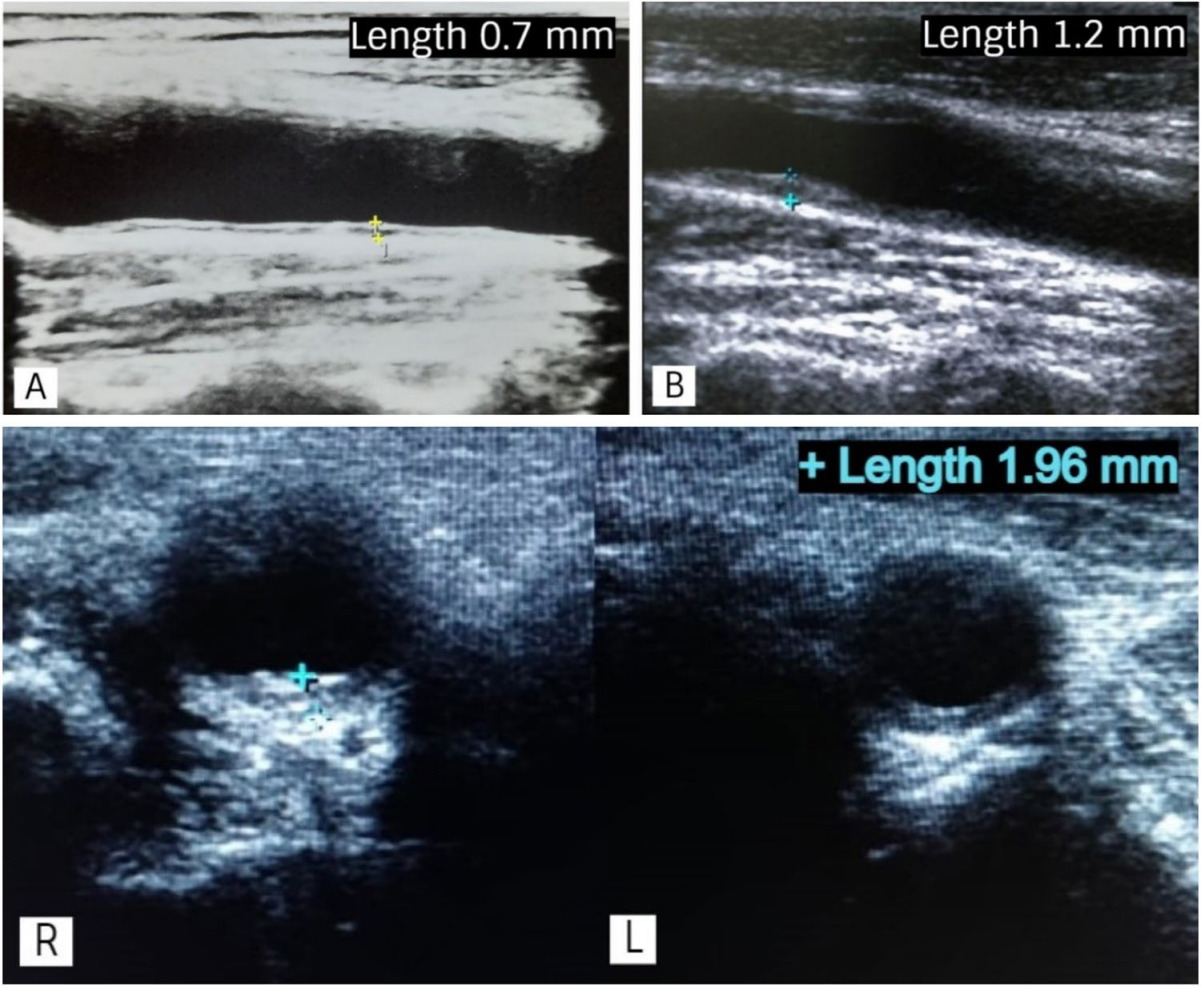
A B-mode ultrasound image (longitudinal section) showing the normal measurement (< 0.9 mm) of carotid intima-media thickness; B increased thickness (> 0.9 mm); C measurement of the carotid intima-media thickness on the right side (axial section); D increased thickness (> 0.9 mm) over the left side (axial section)
